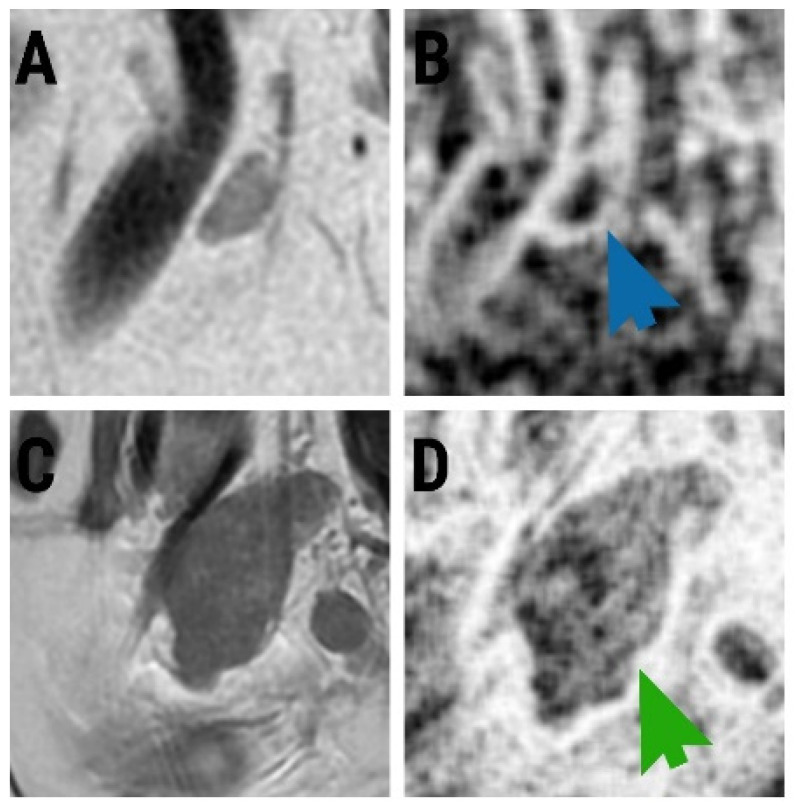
(A) A sagittal T2-weighted image of a non-tumoral lymph node in a 58-year-old patient; (B) generated map of this image based on CZ1S6Entropy parameter (blue arrow pointing to the lesion). (C) A sagittal T2-weighted image of a 61-year-old patient with pathologically confirmed metastatic lymph node; (D) generated map of this image based on CZ1S6Entropy parameter (green arrow pointing to the lesion).
